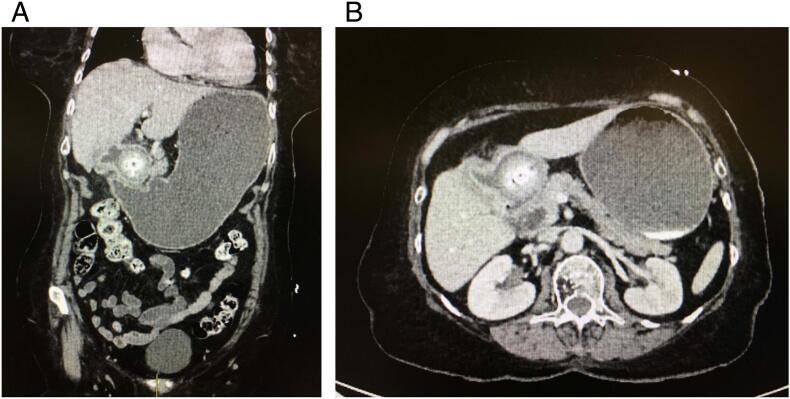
CT scan of abdomen and pelvis showing gastric outlet obstruction secondary to a large gallstone at the duodenal bulb.
